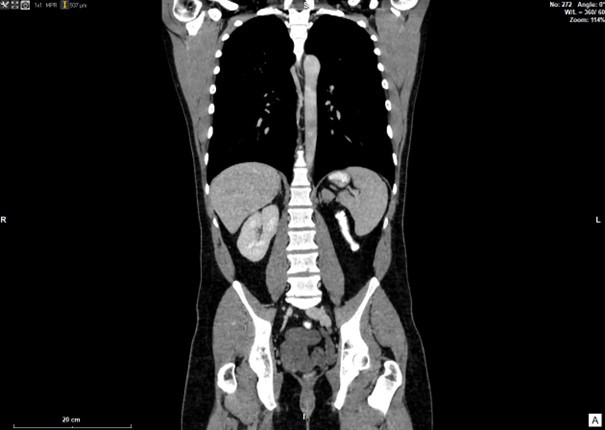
Multi-slice computed tomography with gastrografin showing renal agenesis and a multicystically dilated seminal vesicle.
